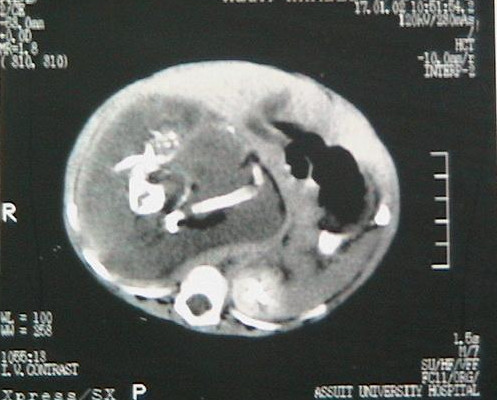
Computed Tomography scan of the patient's abdomen reveals a large retroperitoneal soft tissue mass. There are long hyperdense opacities that resemble fetal bones (see arrows)
